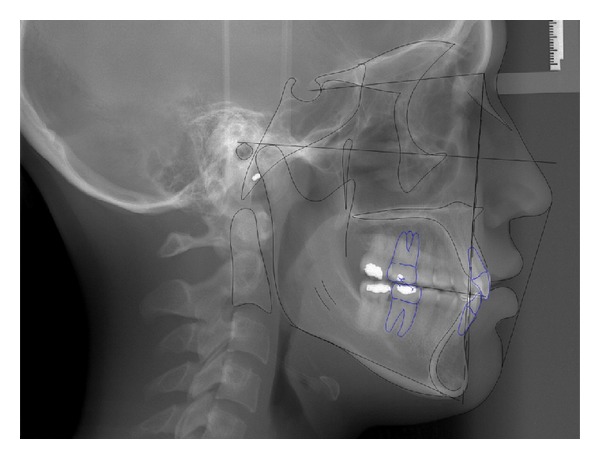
Final lateral cephalometric radiograph.
